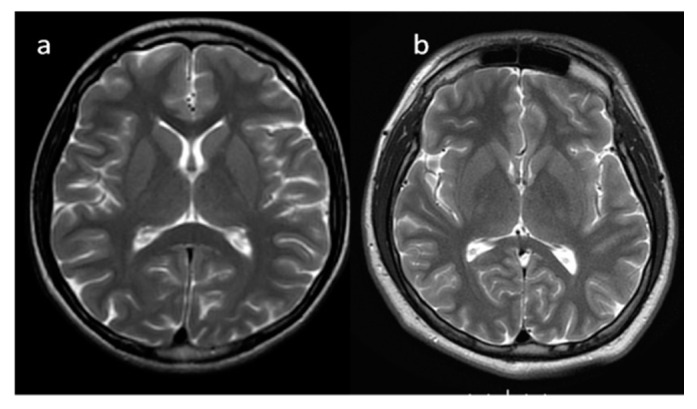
(a,b). Completion of myelination on brain MRI and differences in signal intensity between the basal ganglia and the surrounding brain tissues (axial T2-WI).
